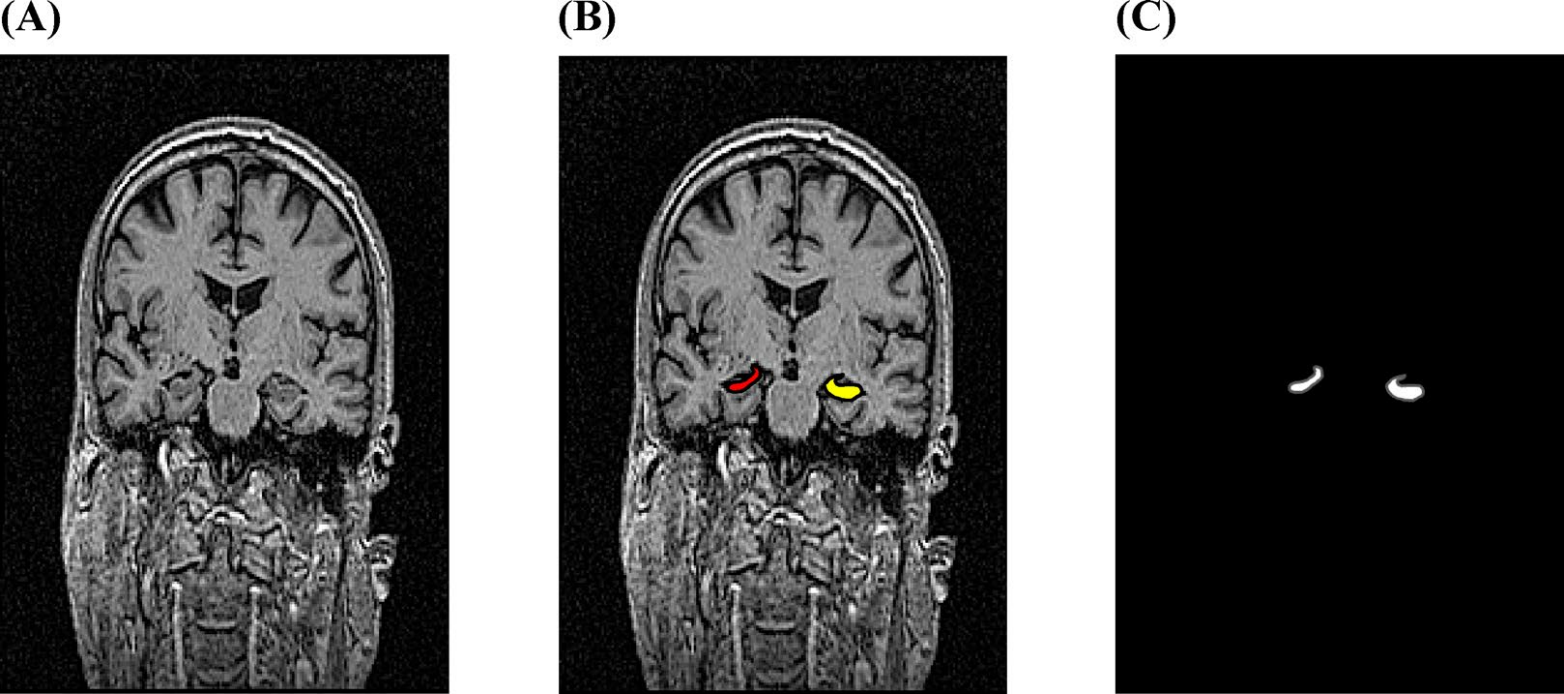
The left and right hippocampus segmentation from the sample MRI images sourced from the Alzheimer’s Disease Neuroimaging Initiative (ADNI) dataset; (a) The input images; (b) The prediction of segmentation images; and (c) The hippocampus segmented area.
